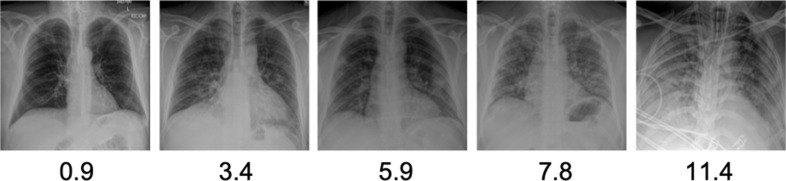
Chest radiograph with their corresponding severity scores from the CNN algorithm. The automated severity score uses a Siamese CNN by using raw DICOM pixel data to calculate a numeric score for lung disease. An increasing score (left to right) corresponds to increasing parenchymal lung opacity and extent. The score is continuous; however, a severity scale proposed from radiologist interpretation is as follows: ≤2.5 no or minimal disease, >2.5 and ≤5.0 mild disease, >5.0 and ≤9.0 moderate disease, and>9.0 severe disease.^
